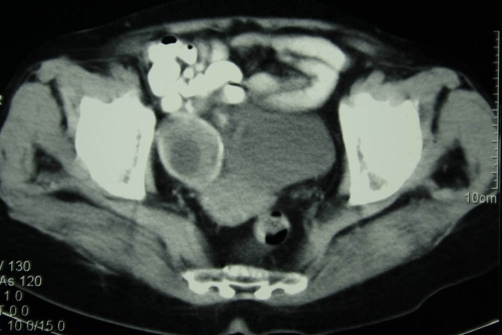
CT section at the level of the pelvis. A second cystic mass completely calcified is present at the right ovary.
